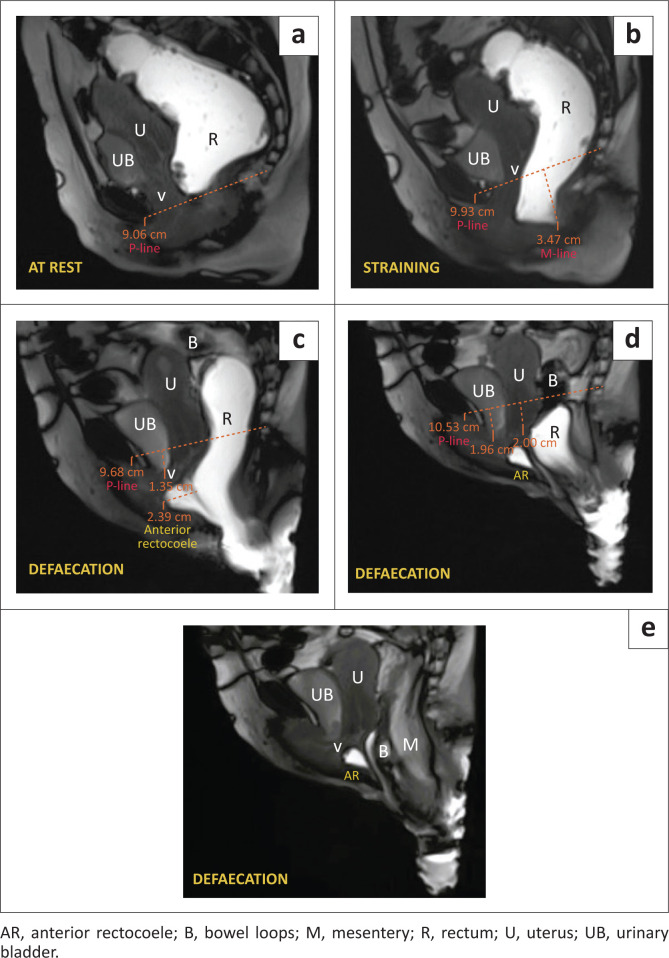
(a–e) MRI defaecogram: Rectal prolapse with enterocoele and anterior rectocoele (a) at rest. (b) Straining phase showing Grade 2 anorectal descent. (c) Defaecation phase depicting medium anterior rectocoele and small cystocoele. (d) Defaecation phase with tricompartment involvement, showing small cystocoele, Grade 1 uterine prolapse, rectum lying below the pubococcygeal line (P-line), bowel loops descending below the P-line and anterior rectocoele with retained jelly. (e) Defaecation phase showing complete rectal prolapse and further descent of the bowel loops (B) and mesentery (M) outside the anal verge.
